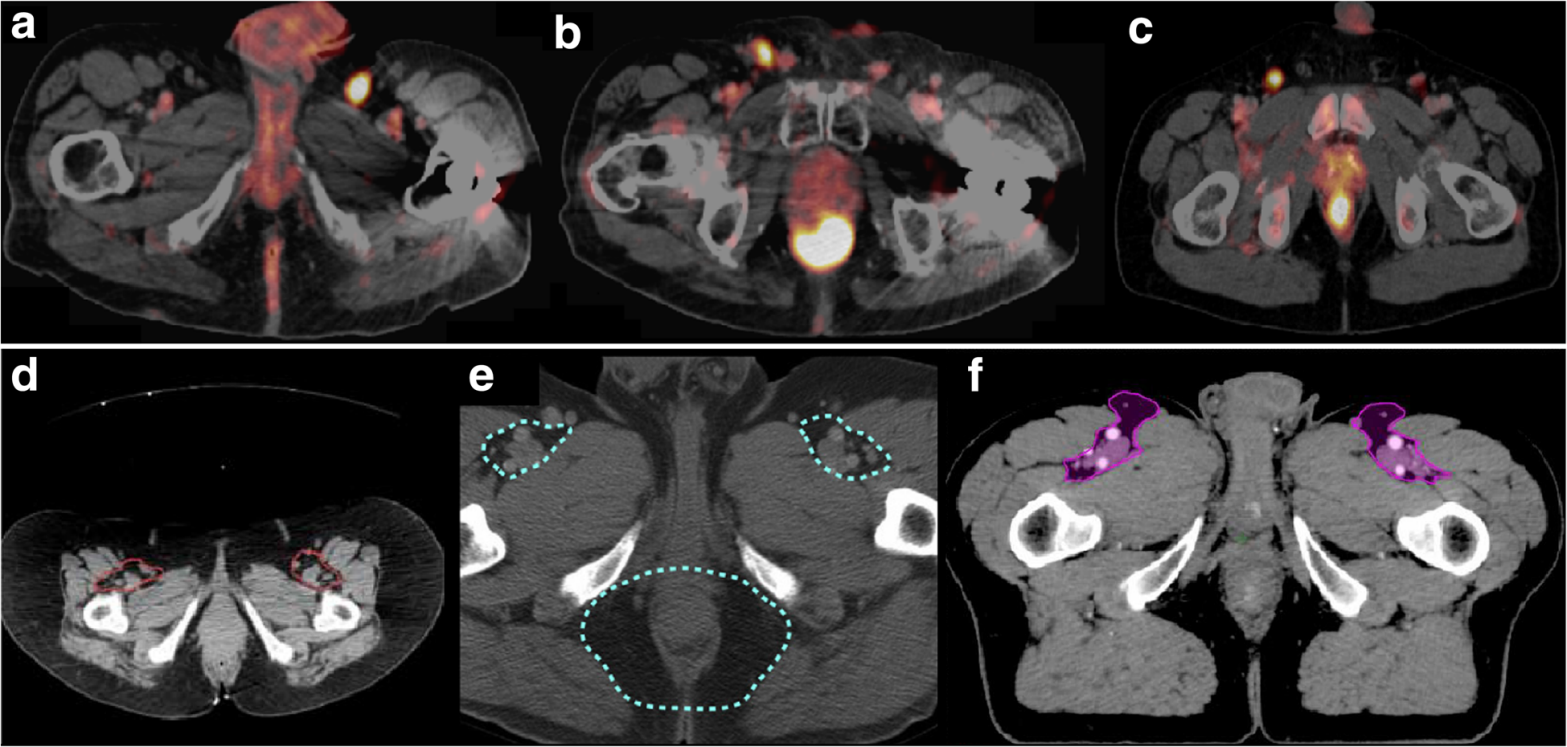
PET-positive superomedial superficial inguinal LN (a–c) in anal cancer patients (SUV_max_ /SUV_mean:_ 3a: 13.3/8.4; 3b: 6.0/3.6; 3c: 5.9/3.4). Those were not properly covered by the elective CTV recommendations of RTOG (d) and AGITG (e) but completely included in the CTV of BNG (f)
